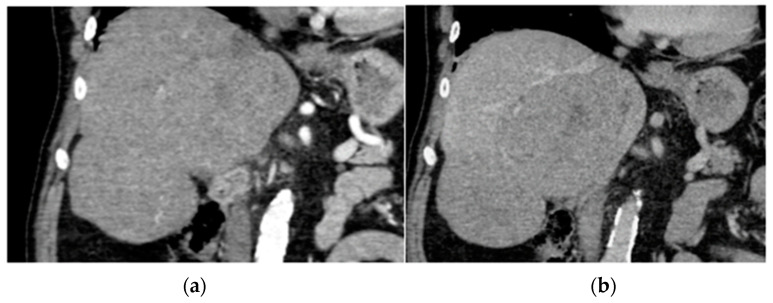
Biopsy-proven Liver EP, case from our institution (a) Arterial phase coronal CT shows two well-defined liver lesions, both of which show mild enhancement; (b) Portal venous phase coronal CT shows a very mild washout of contrast in both lesions.
